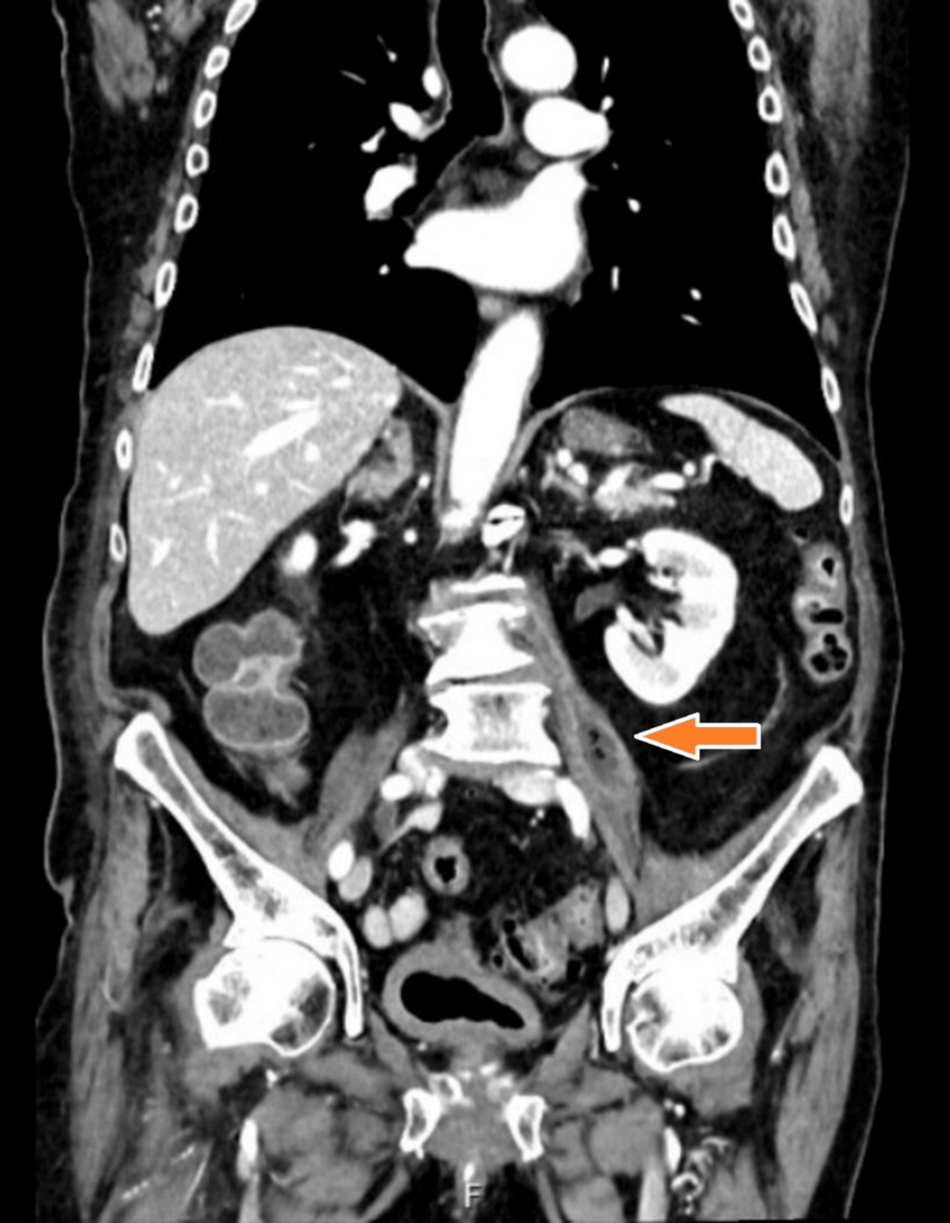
Coronal CT scan of the abdomen and pelvis demonstrating a hypodense, elongated fluid collection within the left psoas major muscle (orange arrow).
